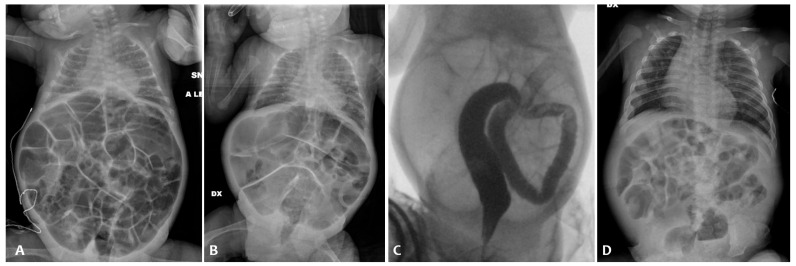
Abdominal X-ray of patient P6 at 34 weeks of postmenstrual age at our NICU admission (A) and after NAC rectal enemas (B); barium enema documenting dolichosigma and intestinal malrotation (C); and abdominal X-ray at discharge (D).
